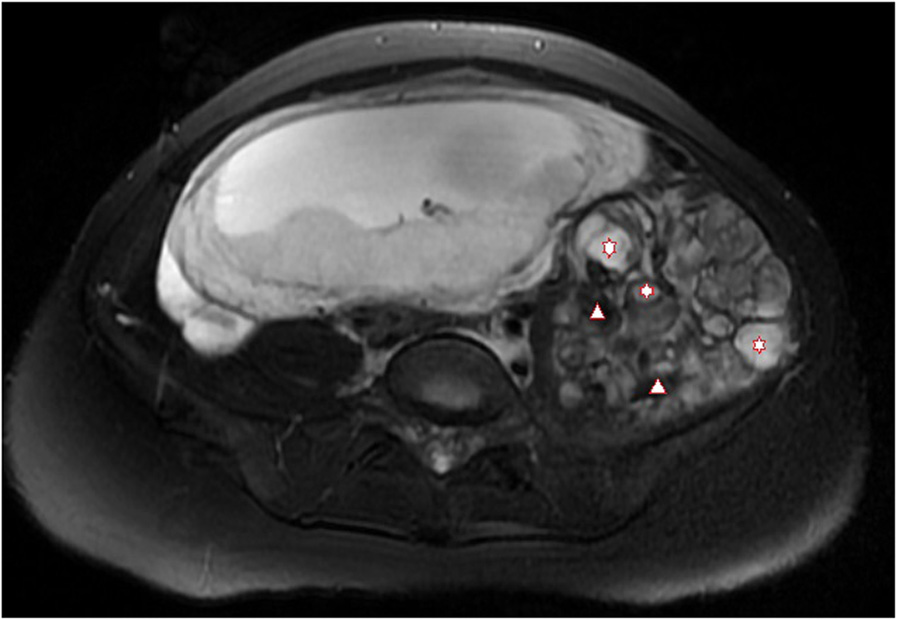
MRI (Axial T2-FRFSE Fat SAT). The well-circumscribed multinodular mass with heterogeneous intensity of this retroperitoneal liposarcoma. Star for tumor, triangle for gelatinous components (2).
